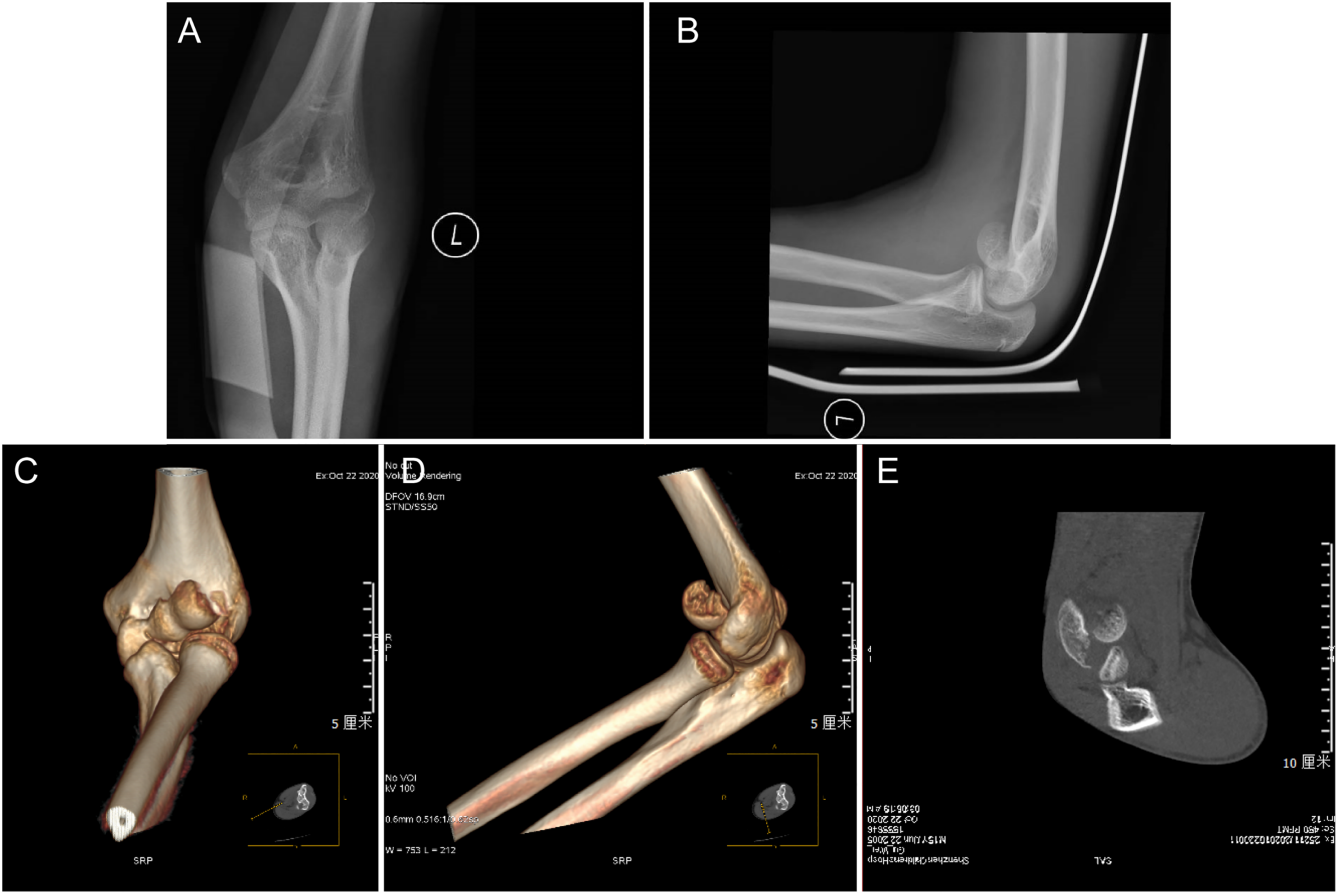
A preoperative radiograph of a 15-year-old boy with capitellum fracture of the humerus.AP (A) and lateral (B). (C–E) The picture shows the CT examination radiographs after injury: CT examination is performed before the operation to show the displacement and relative relationship of the bone fragments.
